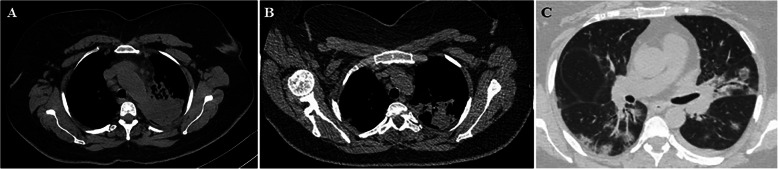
CT imaging findings; a A mass like lesion was shown in the left upper lobe apicoposterior segment. b Dimensional regression of the mass like lesion and a consolidated area containing air bronchograms was observed in the left upper lobe apicoposterior segment 6 days later. c Follow-up CT scan 6 days later showed typical COVID-19 findings with multifocal segmental ground glass opacity infiltration and consolidation with air-bronchogram along the bronchovascular bundles and in subpleural areas with associated interlobular septal thickening and minimal pleural effusion in the left lower lobe
